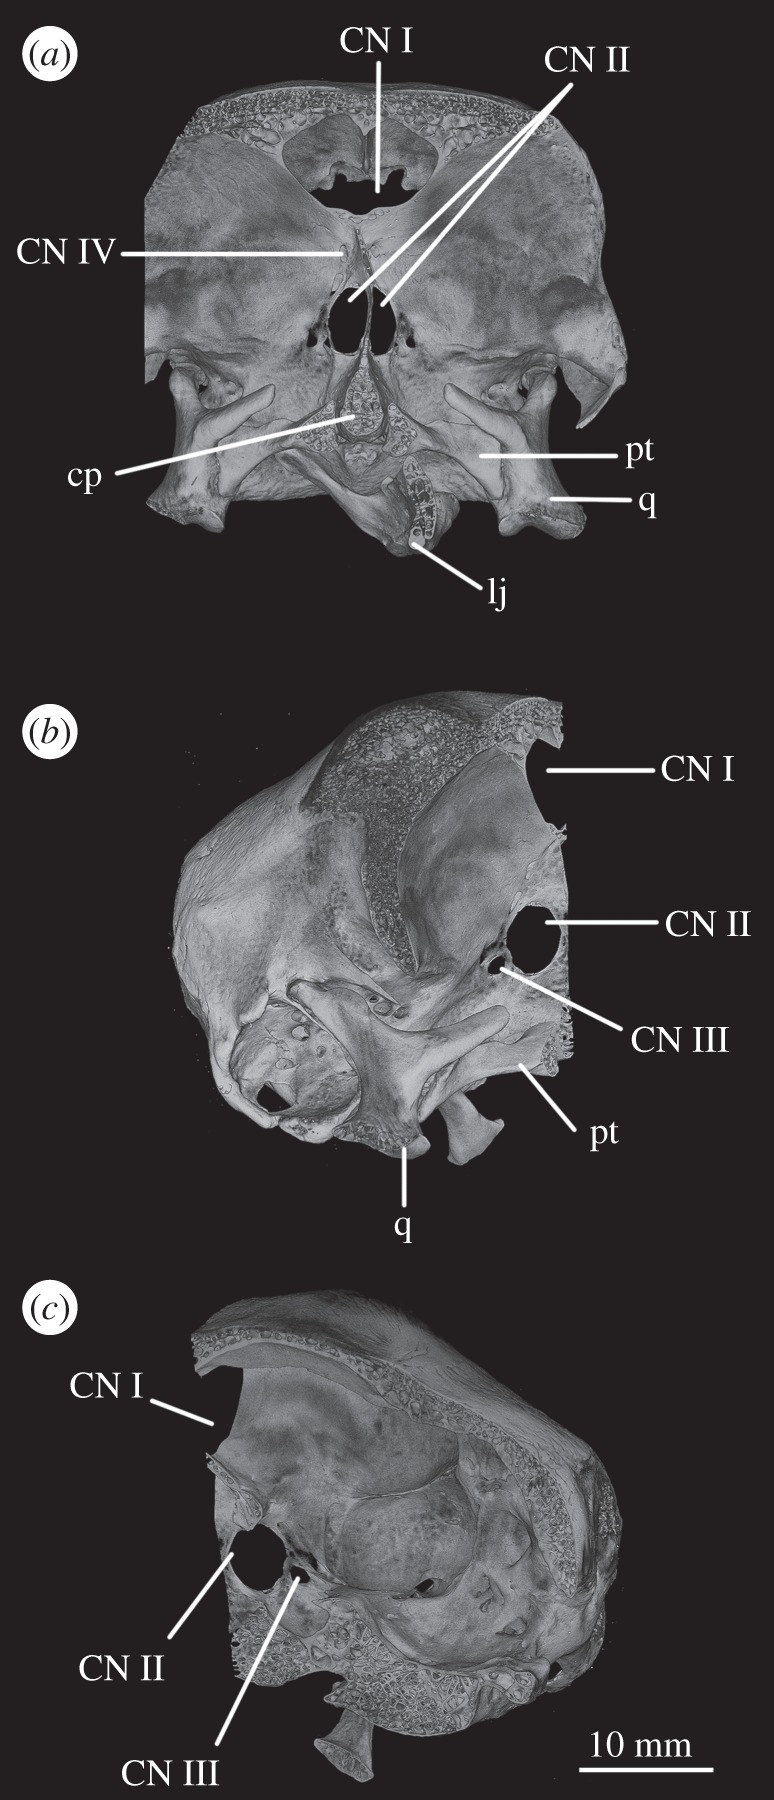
CT reconstructions of the braincase of *Meleagris gallopavo* (ZMB 1793 792) in (*a*) anterior, (*b*) right anterolateral, and (*c*) left medial views. For abbreviations, see table 1.
